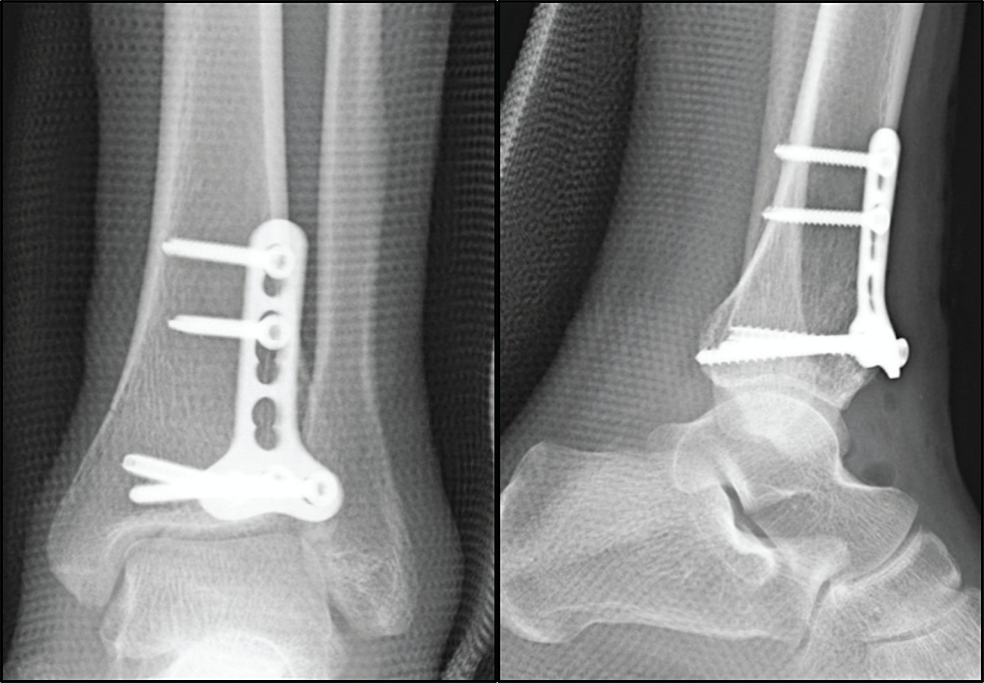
Postoperative radiograph showing the fixation and immobilization with plaster splint
